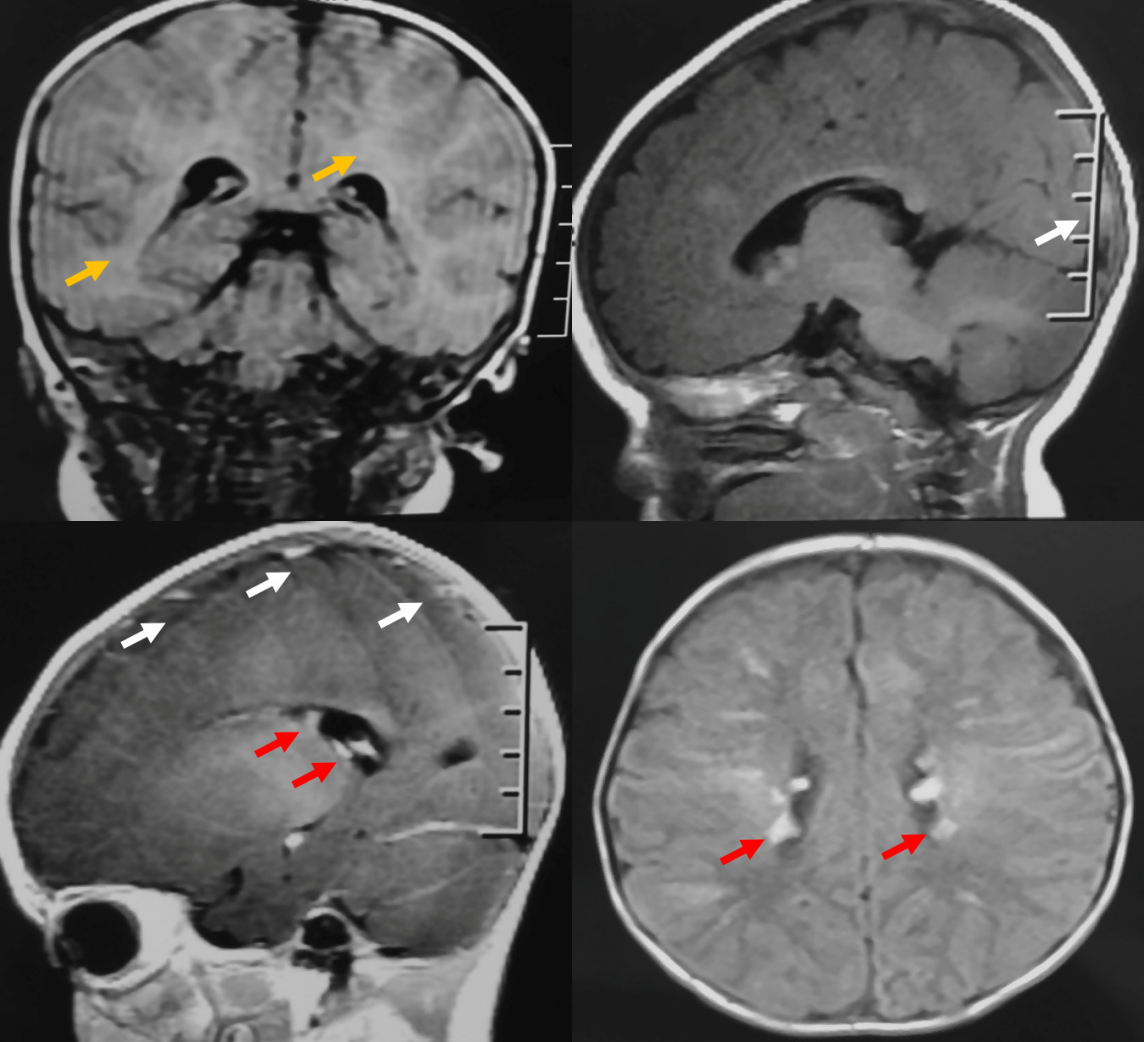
Cerebral MRI revealing diffuse areas of commitment in the white matter (yellow arrow) and cortex with multiple frontal, temporal, parietal, and occipital (white arrow), subependymal lesions of tubers (red arrow).
